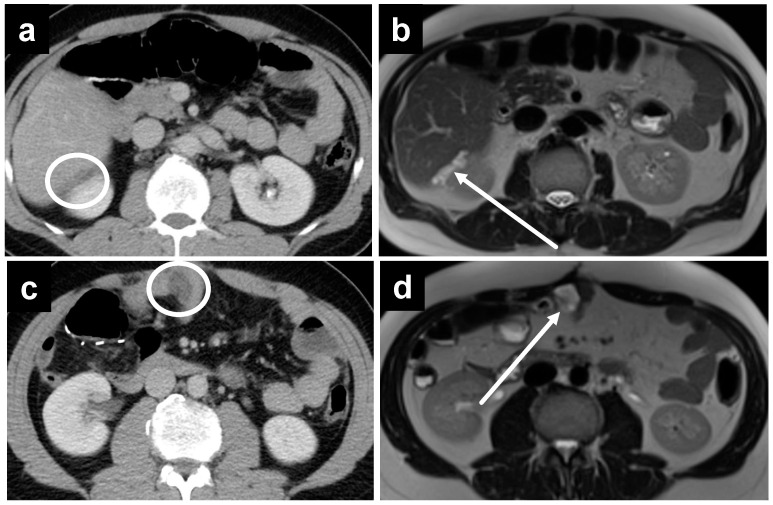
A 44-year-old male with recurrent mucinous appendiceal tumor; the hypodense fluid is not well seen on the (a) CT at Morrison’s pouch but easily seen on the (b) T2W MRI, where the fluid also appears to be loculated, suggestive of a tumor deposit as opposed to bland ascites. Similarly, the pocket of fluid among small bowel loops is not as well appreciated on (c) CT but is well seen on (d) T2W MRI.
